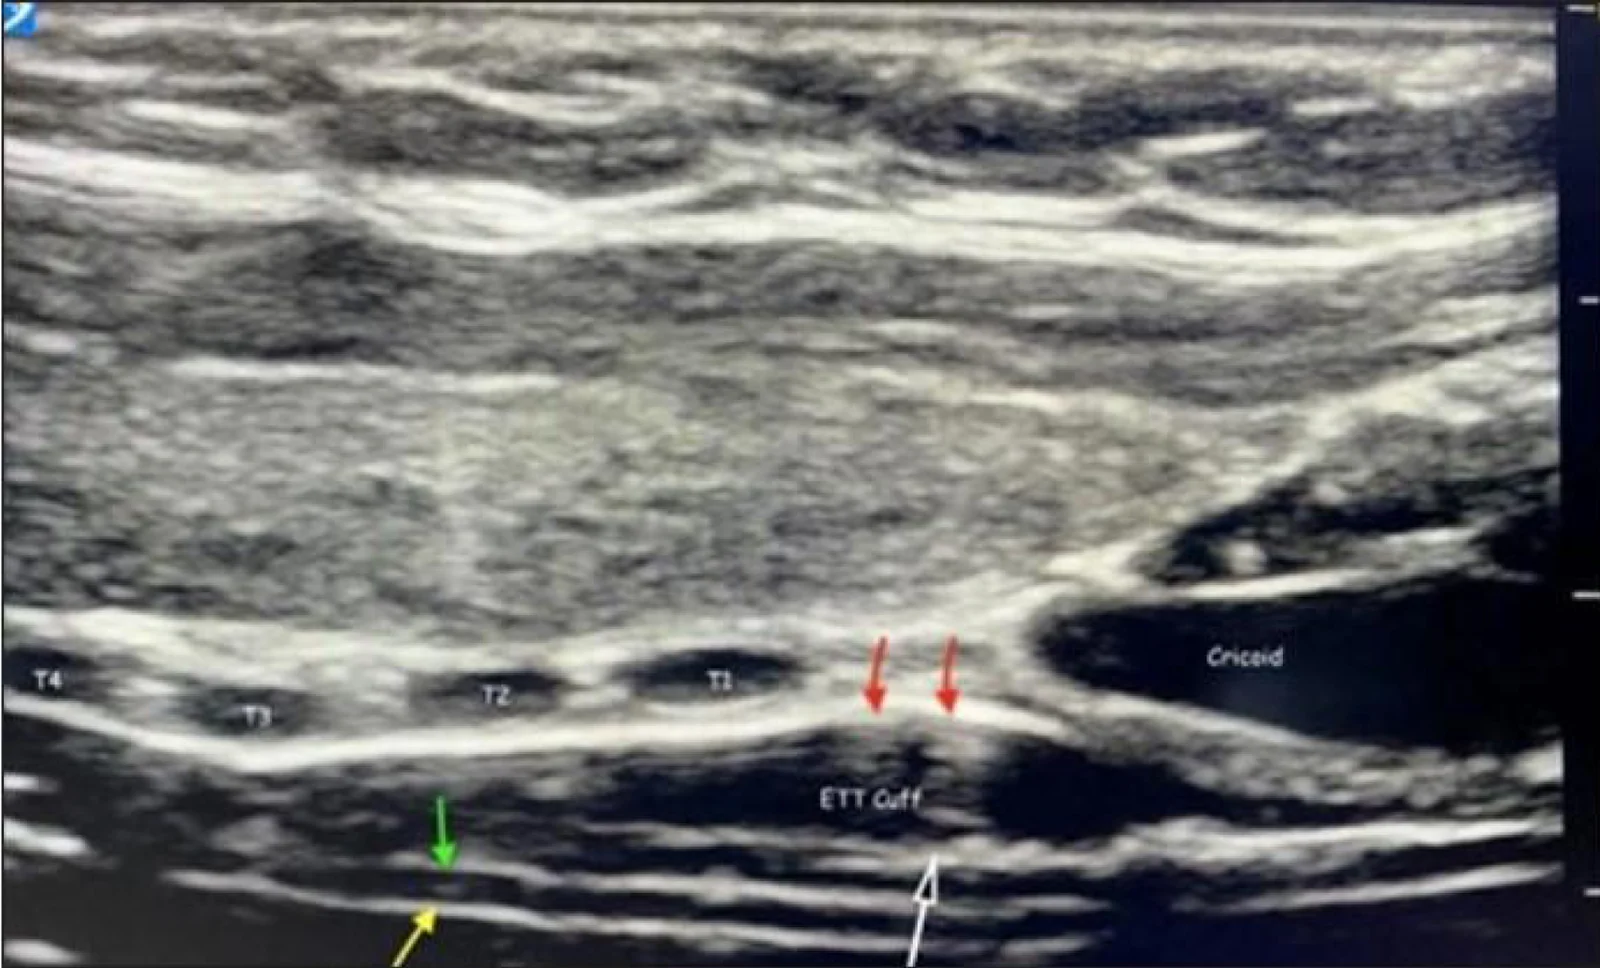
Ultrasonographic images of the airway and endotracheal tube (ETT). The cricoid is an anechoic structure to the right and the tracheal rings are numbered (T1 to T4). To enhance visibility on ultrasound, the cuff of the ETT is filled with saline (white arrow). The cephalad aspect of the cuff is positioned at the lower aspect of the cricoid. A linear hyperechoic line (red arrows) on the longitudinal plane of the trachea is formed by reverberation artifacts from the air-mucosa interface within the trachea (red arrows). The walls of the ETT within the trachea are seen as two hyperechoic lines (yellow and green arrows).
